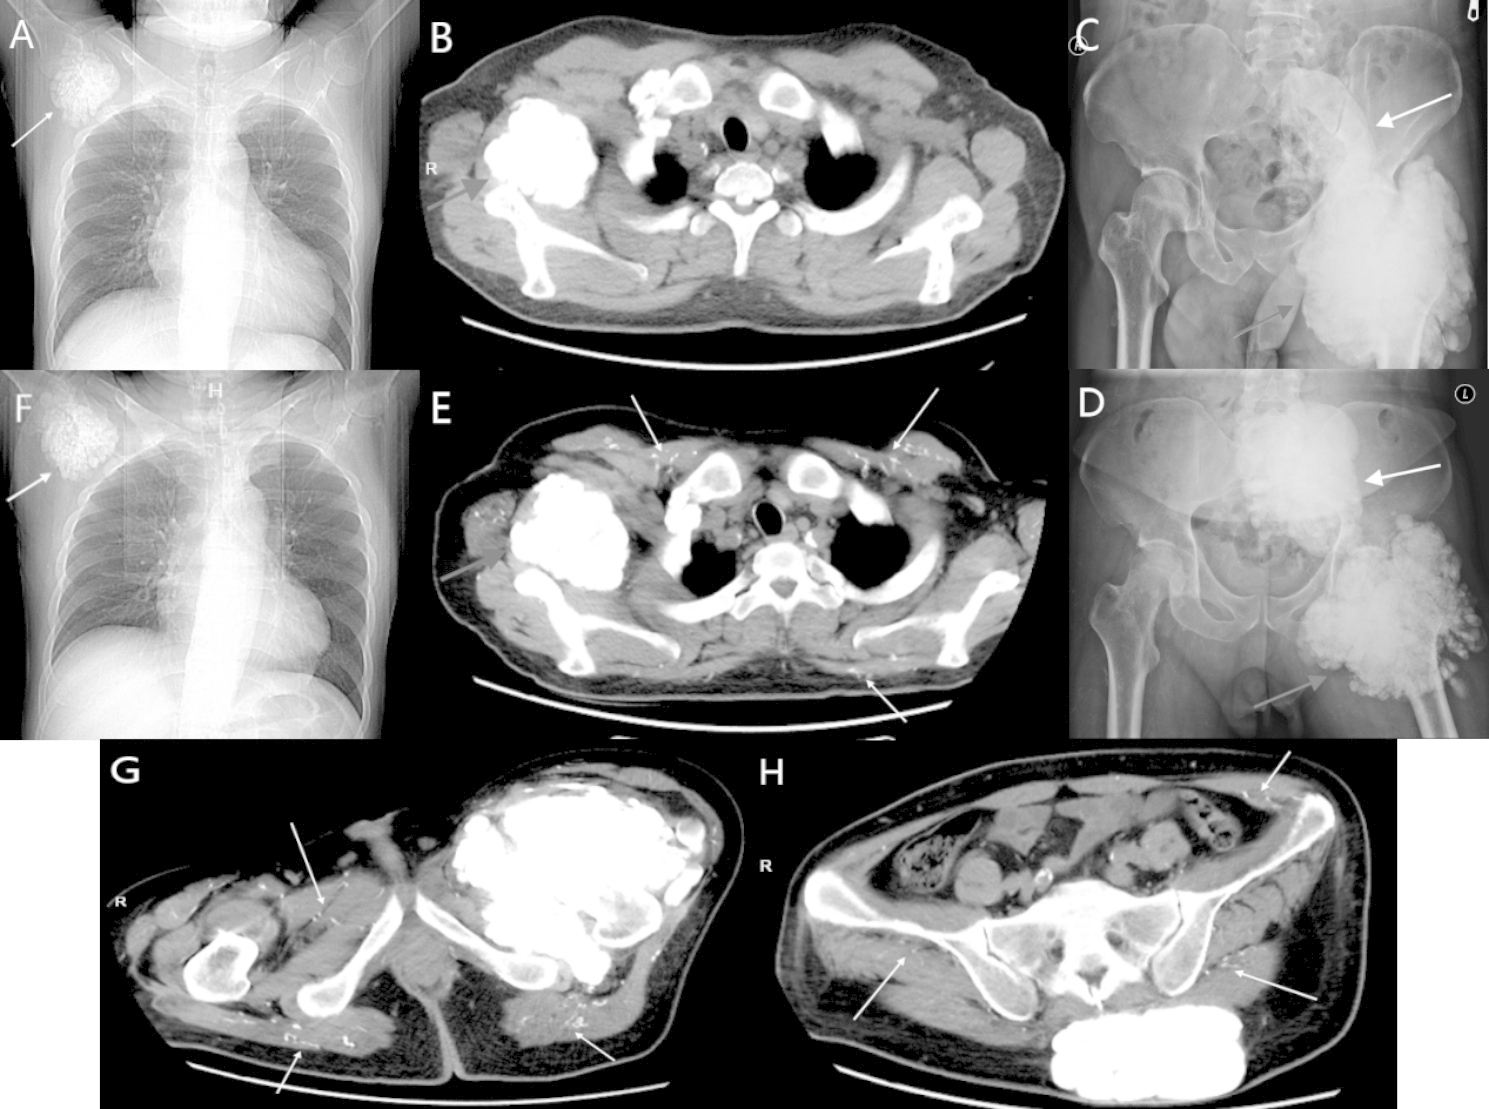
Standard X-ray shows the masses in the shoulder (3 A, 3B), the left hip joint (red arrow in 3 C) and the pelvis (white arrow in 3 C) before parathyroidectomy in case 2. Nineteen months after parathyroidectomy, the masses in the shoulder (3E, 3 F), the left hip joint (red arrow in 3D), and the pelvis (white arrow in 3D) in case 2. CT scan of shoulder joint calcification, shoulder joint (red arrow in 3E) and vascular calcium (3E, 3G-3 H) 19 months after parathyroidectomy in case 2
